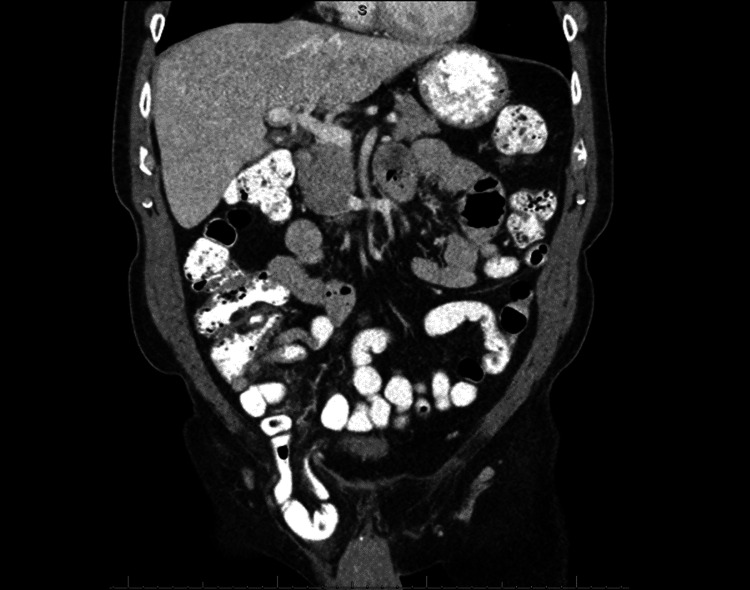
CT showing known right inguinal hernia with the loop of small bowel CT: computed tomography
